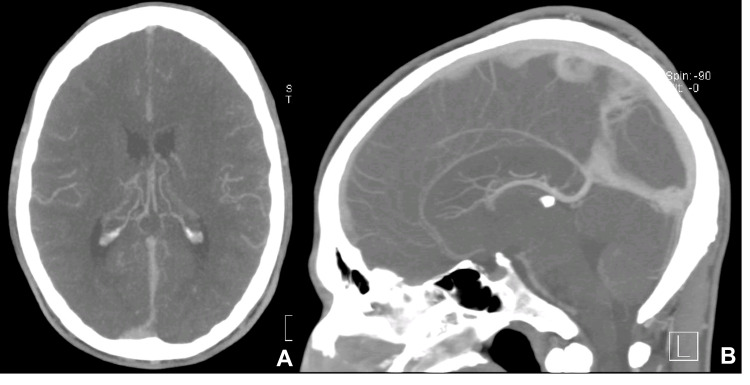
CT cerebral venous angiography without changes CT: Computed tomography (A) Axial CT cerebral venous angiography section. (B) Sagittal CT cerebral venous angiography section
